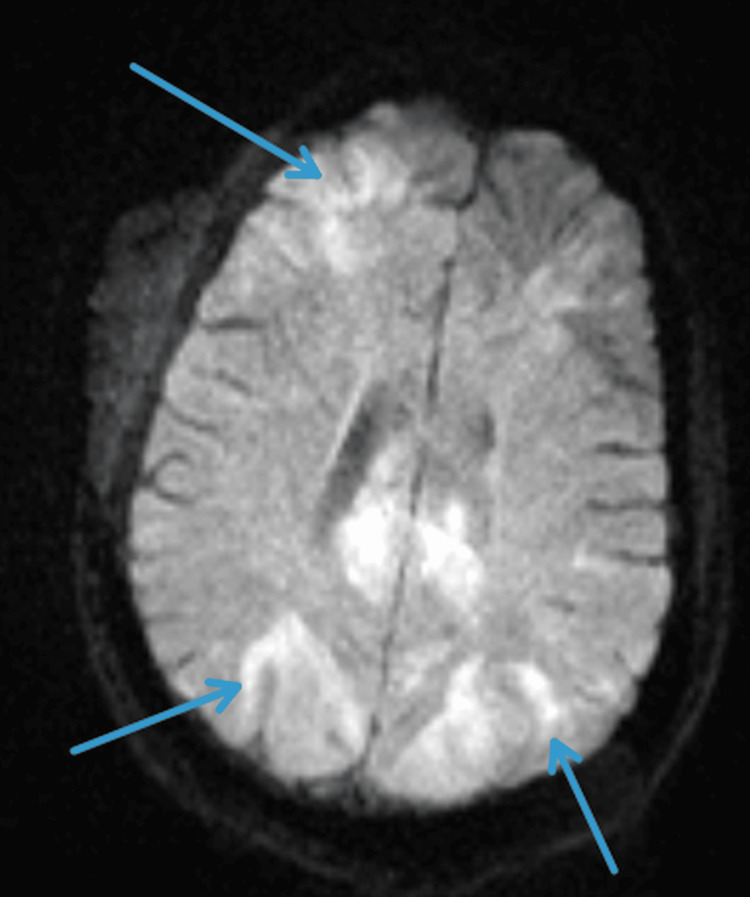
Axial diffusion-weighted MRI demonstrating multifocal areas of diffusion restriction consistent with cytotoxic edema and active inflammatory involvement.
